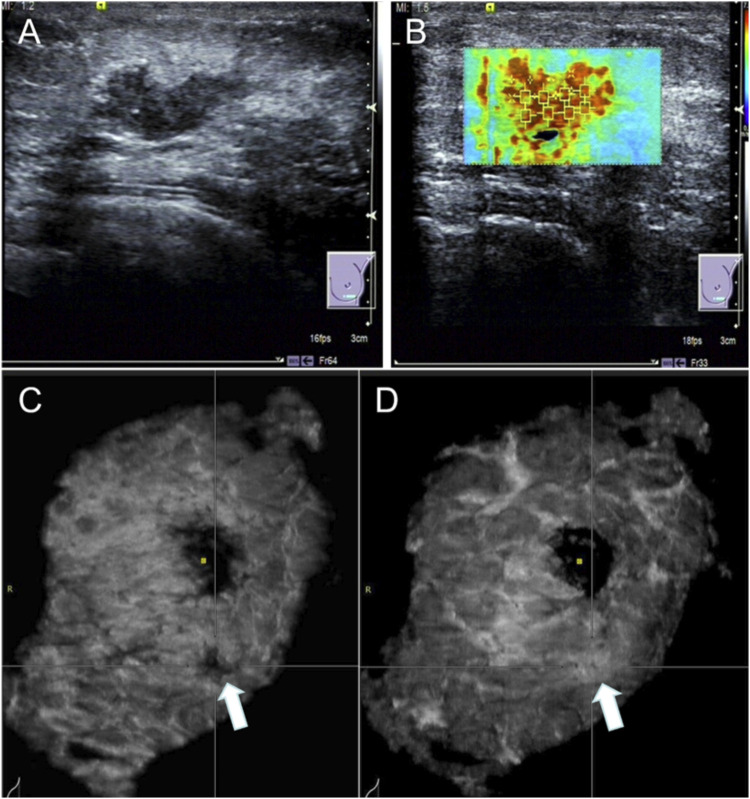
Ultrasound images of a case involving a 63-year-old woman with a lesion measuring 605015 mm in diameter. Pathology results: malignant tumor in the left breast. (A) conventional 2D ultrasound image; (B) VTIQ average measurement: 6.89 m/s; (C) ABVS coronal view showing the mass (arrow); (D) “cloud sign” (arrow).
